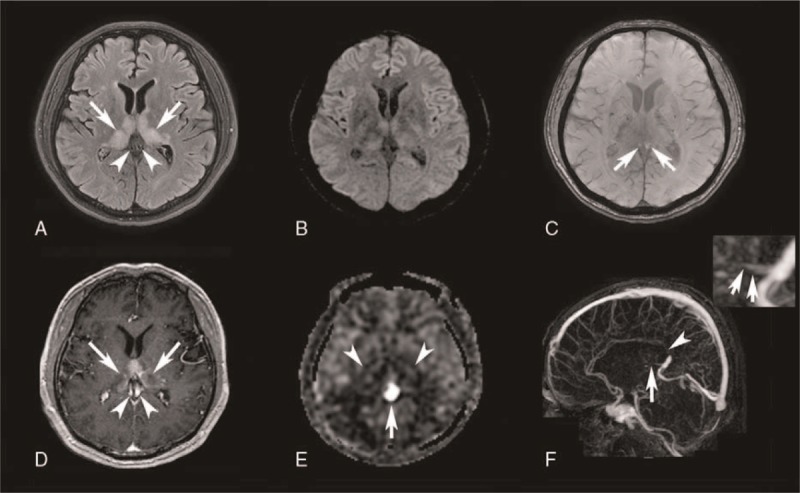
A 47-year-old man presented with memory disturbance and confusion for 2 weeks. (A) Fluid-attenuated inversion recovery image demonstrates hyperintense lesions in the bilateral thalami (arrows) and preserved signal void in the internal cerebral veins implying inner blood flow (arrowheads). (B) No definite abnormal focus with diffusion restriction is noted on diffusion-weighted image. (C) Susceptibility vessel sign is not on susceptibility-weighted image (arrows). (D) Contrast-enhanced T1-weighed image shows enhancement in the bilateral thalami suggestive of subacute stage infarct (arrows). However, empty delta sign in the internal cerebral veins is not definite (arrowheads). (E) On arterial spin labeling image, bright sinus appearance is apparent at internal cerebral veins (arrow). Hypoperfusion in the bilateral thalami is also noted (arrowheads). Bright sinus appearance was also apparent at inferior sagittal sinus (not shown). (F) Four-dimensional CT venography revealing filling defects in the bilateral internal cerebral veins at distal portions (arrow in the raw image and arrows in the zoomed image). Another filling defect is noted at inferior sagittal sinus at distal portion (arrowhead).
